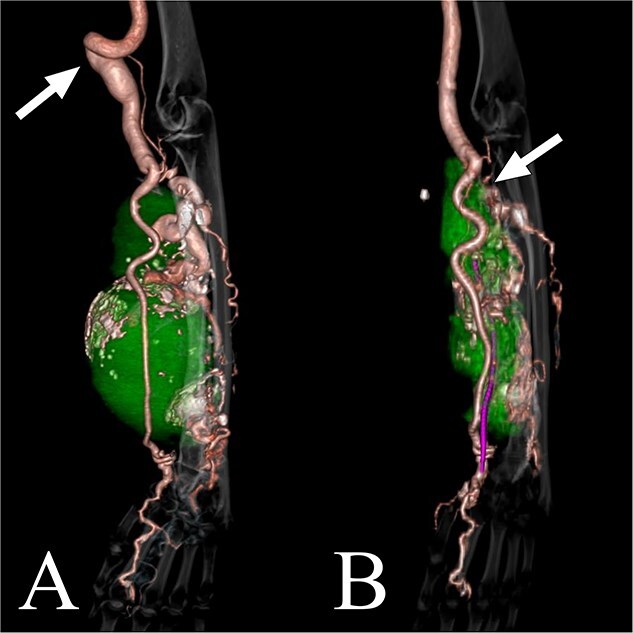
Three-dimensional CT angiography before and after definitive surgical intervention. (A) Preoperative imaging. The arrow indicates the markedly tortuous segment of the brachial artery, which served as the origin of collateral circulation to the aneurysm. (B) Postoperative imaging following endoaneurysmorrhaphy. The arrow indicates a residual collateral vessel, suggesting incomplete occlusion of an inflow source. The purple structure represents the drain inserted into the aneurysmal sac.
